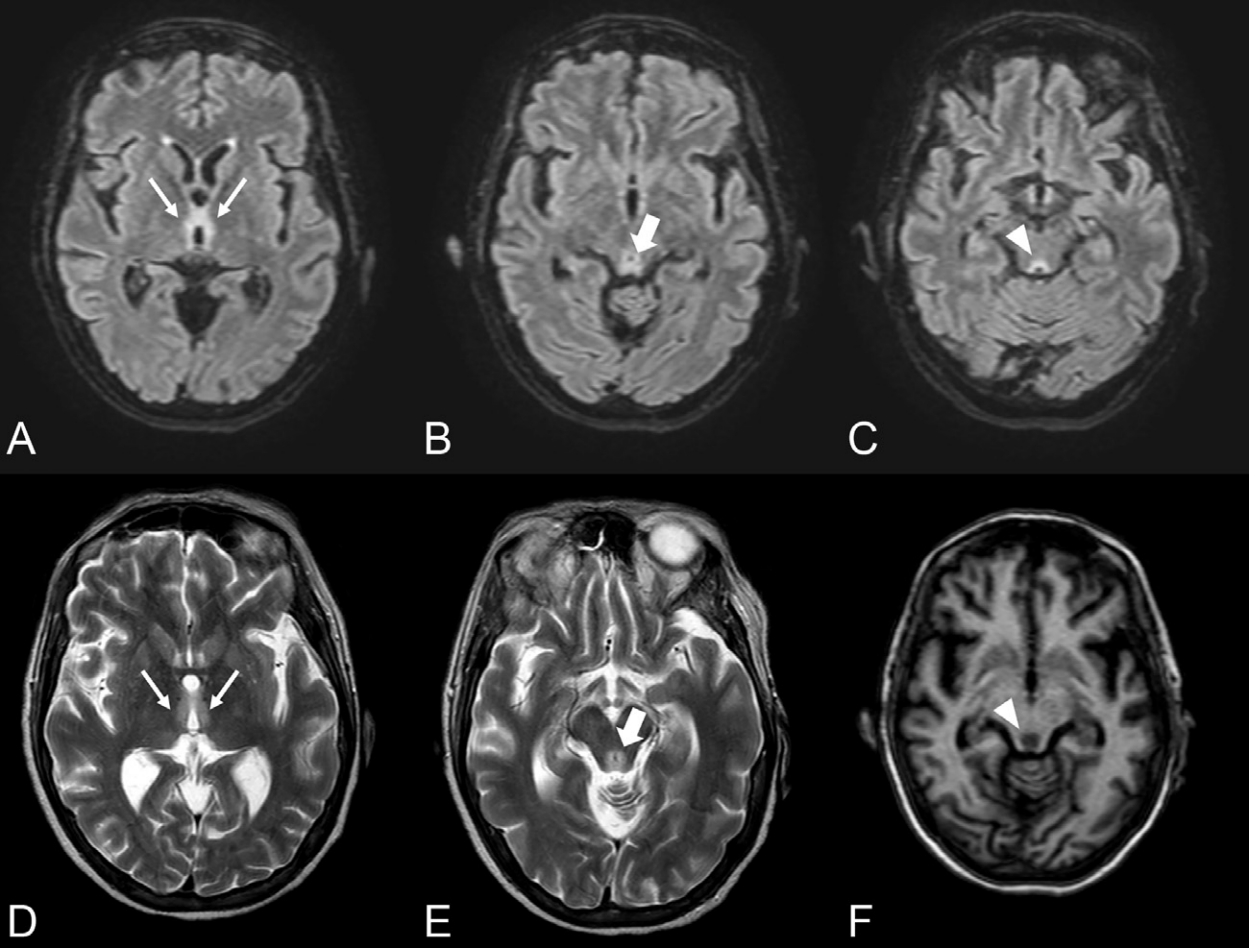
Axial fluid attenuated inversion recovery (FLAIR) (A,B,C), T2-weighted (D,E). High signal intensity is observed in both medial thalami (thin arrow in A and D), in the tectal plate (arrow in B and E) and in the periaqueductal region (arrowhead in C). T1-weighted image (F) shows low signal intensity in the periaqueductal region.
